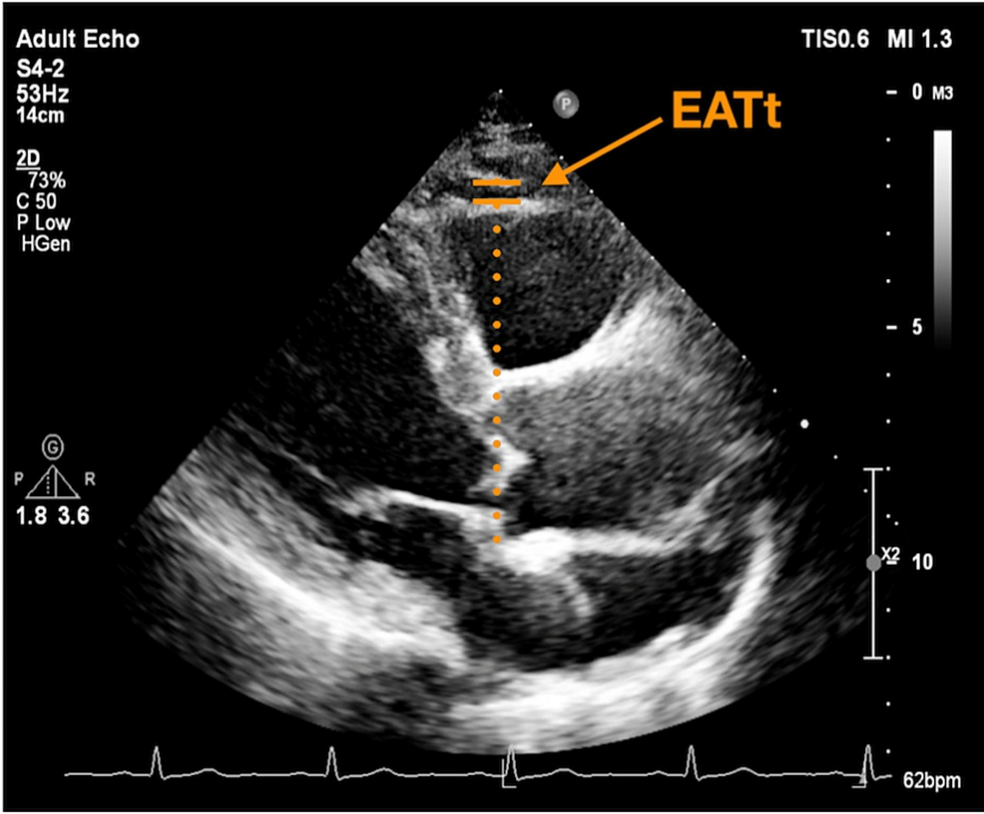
How to measure epicardial adipose tissue thickness (EATt) from the parasternal long-axis view
